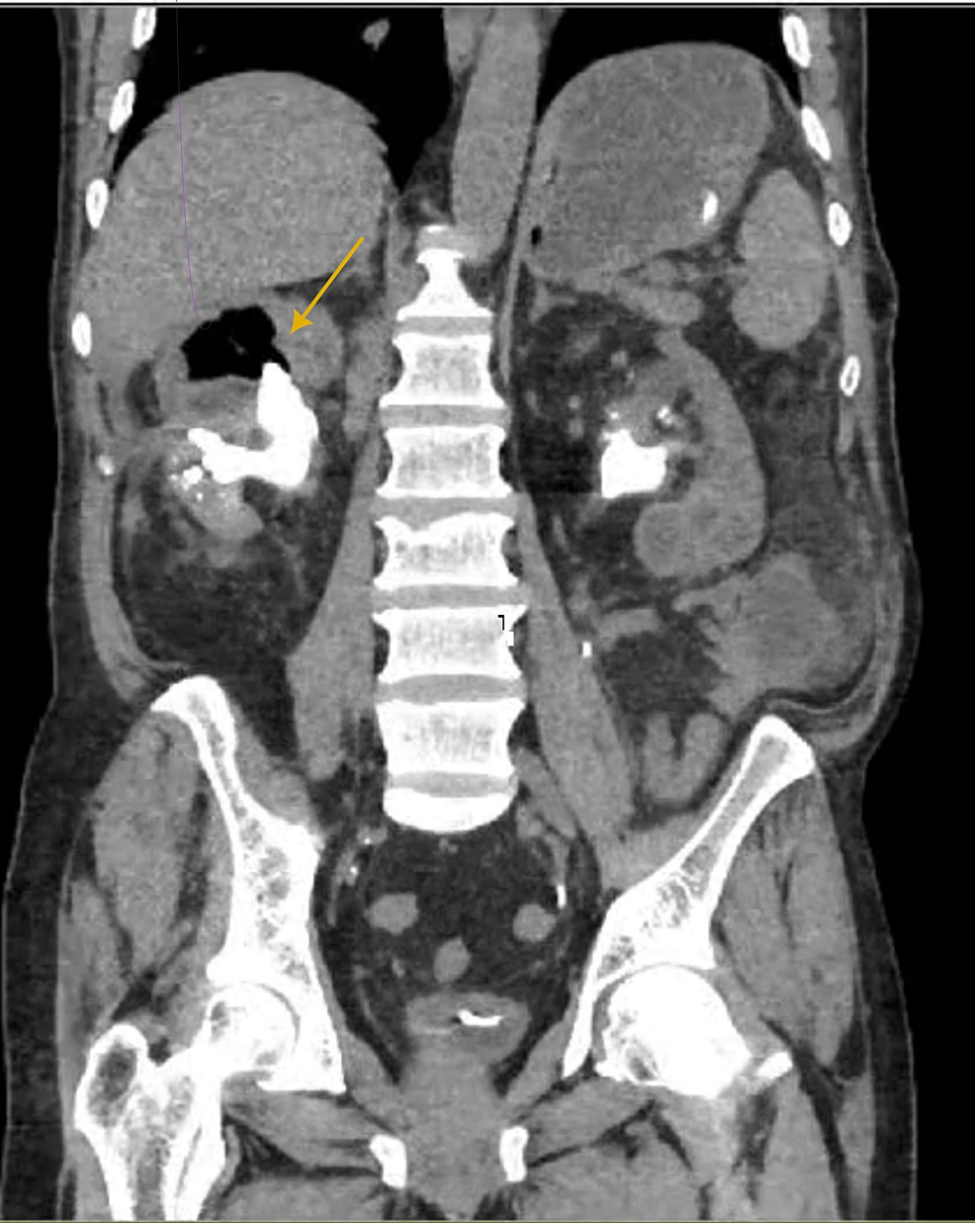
Coronal plane CT KUB show the presence of bilateral staghorn stones and right emphysematous pyelitis
